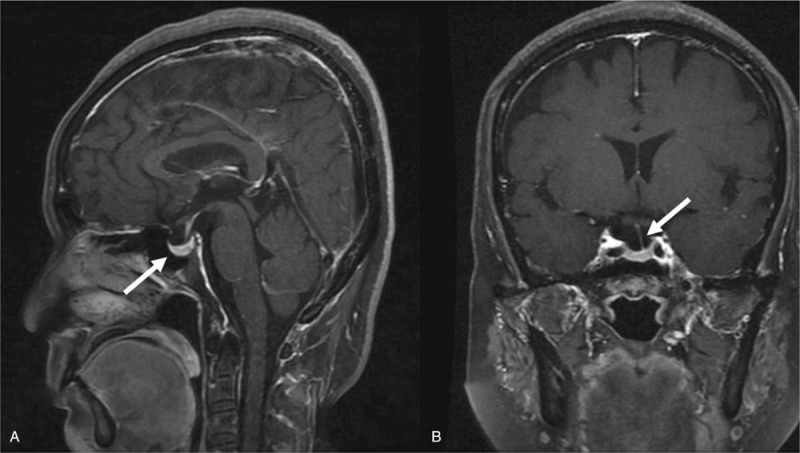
Magnetic resonance images. (A) T1-weighted image in sagittal section view shows that the pituitary gland is pressed downwards, and the thickness is about 0.4 cm (white arrow). (B) T1-weighted image in coronal section view shows that the pituitary stalk is shifted to the left (white arrow).
